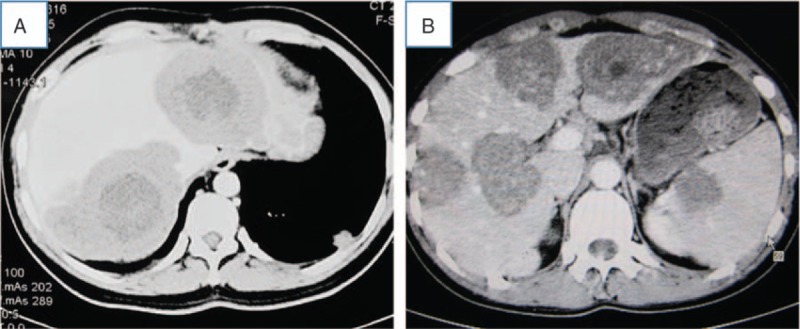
Representative abdominal computed tomography images of alveolar echinococcosis. (A) Abdominal computed tomography showing a patient with two huge alveolar echinococcosis lesions in the right and left lobes. (B) Abdominal computed tomography scan showing a patient with 4 big liver lesions and a metastatic lesion in spleen.
